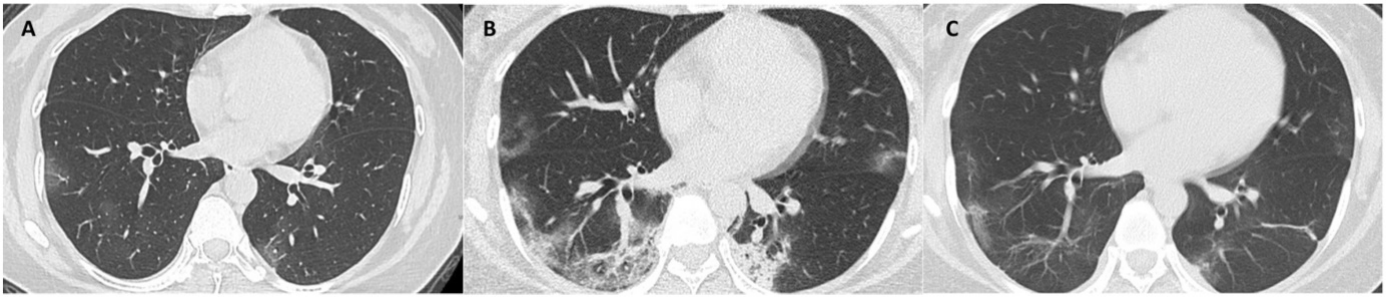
CT scans at 3 milestones in a 33-year-old woman with COVID-19 pneumonia. (A) Initial CT obtained on illness days 7 showed ground-glass opacity in bilateral lower lobe. (B) Worst CT obtained on illness days 13 showed ground-glass opacity and consolidation with largely increased extent in bilateral lower lobes. (C) CT scan before discharge obtained on illness days 22 showed mild residual ground-glass opacity. The patient was discharged on illness days 25.
